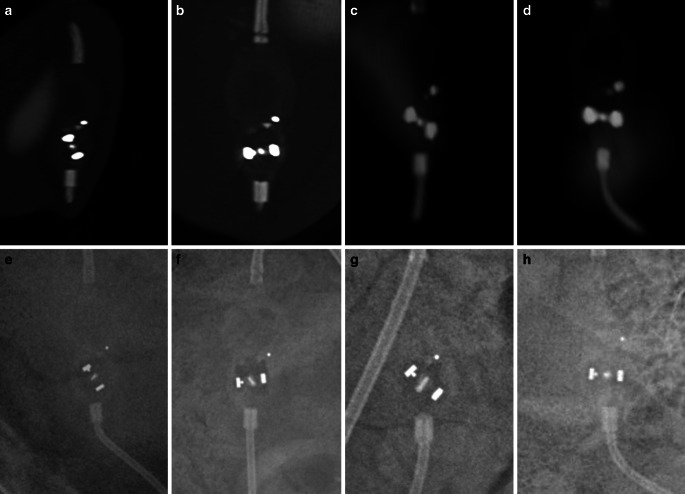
Comparison of Codman Certas VP shunt valves in CT MIP reconstructions using photon-counting CT (a, b) and third-generation CT (c, d), compared with lateral skull radiographs. The valves are shown at pressure settings 5, 4, 5, and 4 (from left to right)
